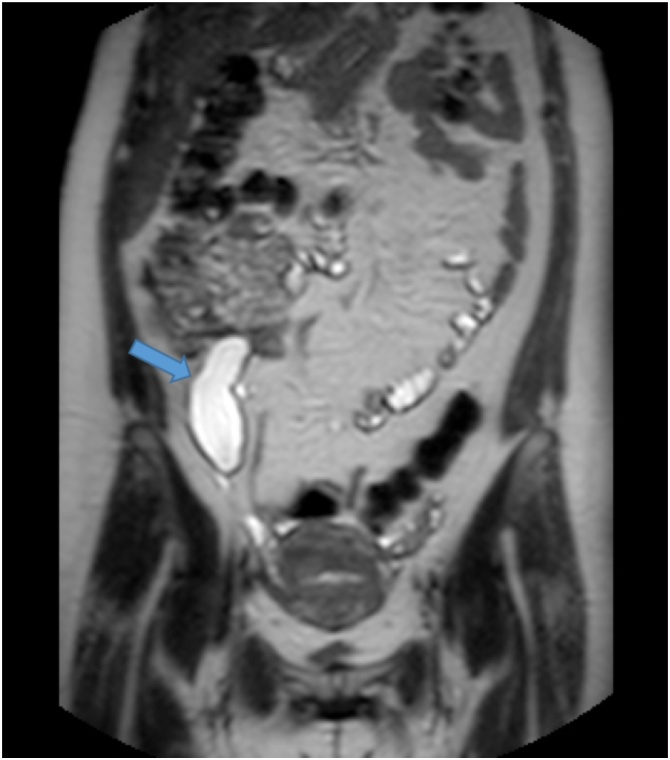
MRI of the abdomen showed: Sizable cyst-like measuring 7 × 4 × 3 cm in the right iliac fossa region, very close to the cecum and away from the right ovary and adnexa.
